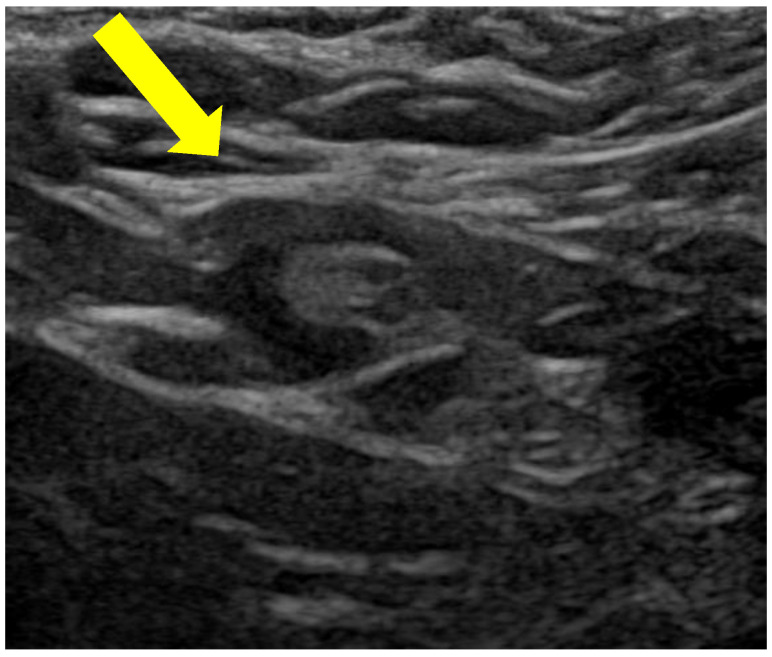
Surface ultrasound echography examination revealed the swollen axillary lymph node, enlarged to a diameter of 15 mm (yellow arrow).
